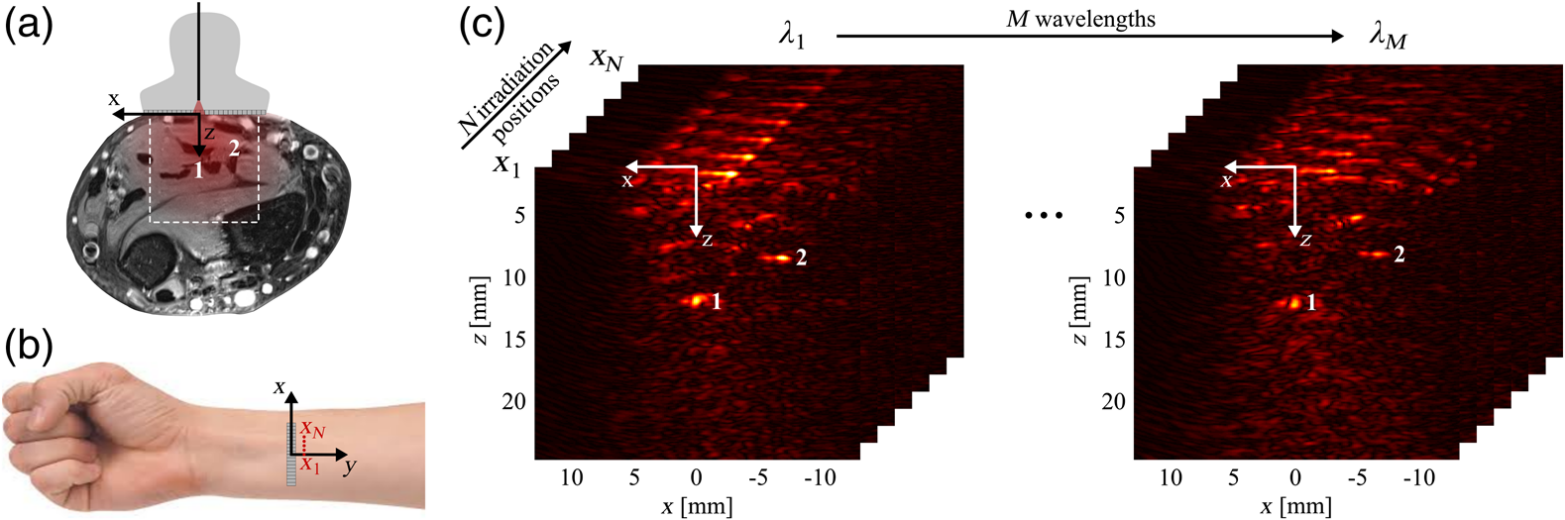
(a) Sketch (not to scale) of the illumination fiber and US probe on a cross-sectional magnetic resonance image of the volunteer’s forearm, displaying the position of the vessels of interest, namely (1) the median artery and (2) a side branch of the median antebrachial vein. (b) Orientation of the linear US transducer array and the irradiation spot, which is translated along x (not to scale). (c) One data set is formed by N·M reconstructed OA images $S_{ij}(r)$, the OA signals of the two vessels of interest are clearly visible.
